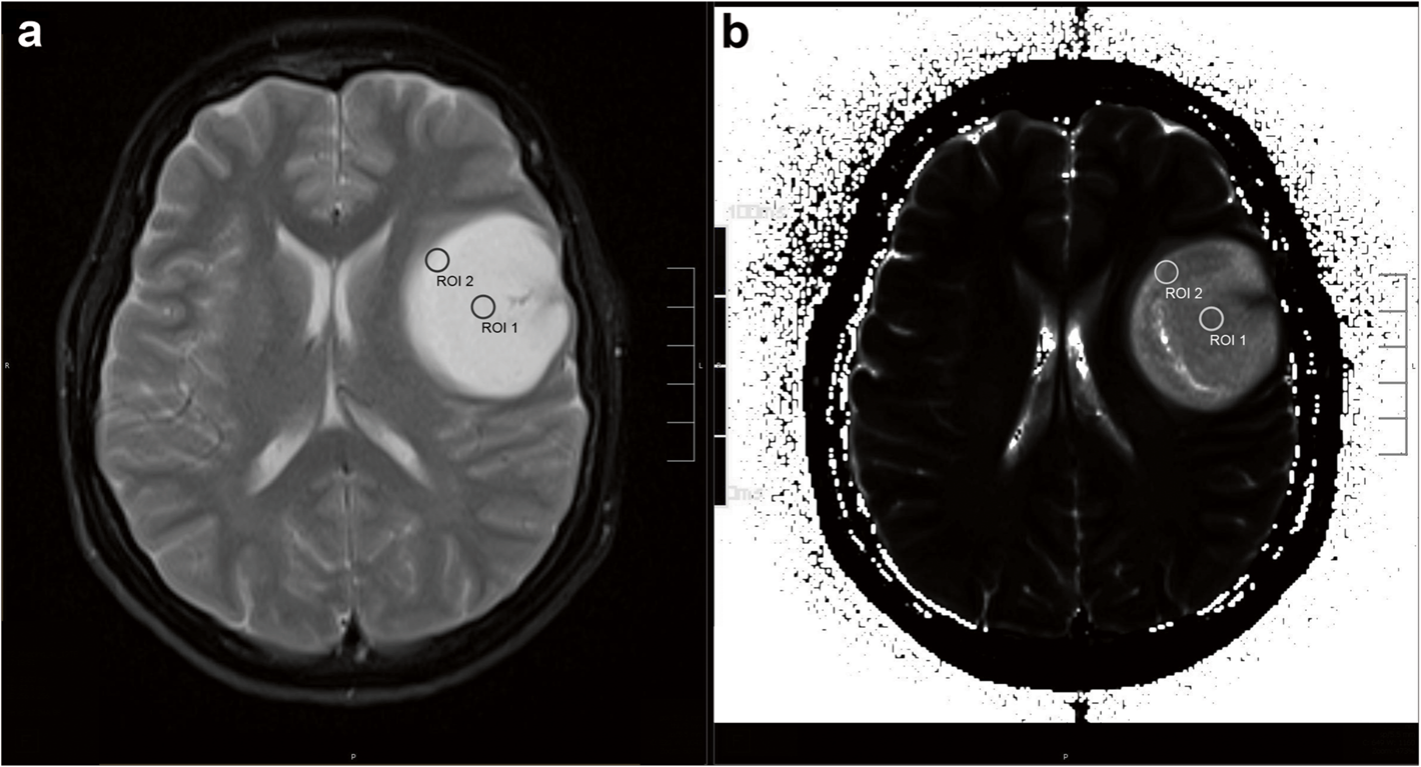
MRI of a 32 years old female patient suffering from IDH-mut anaplastic astrocytoma WHO grade III. The T2 hyperintense tumor shows no enhancement or necrosis and only small edema can be assumed. The central ROI and peripheral ROI are delineated on the transversal T2 image (a) and on the corresponding T2 map (b)
